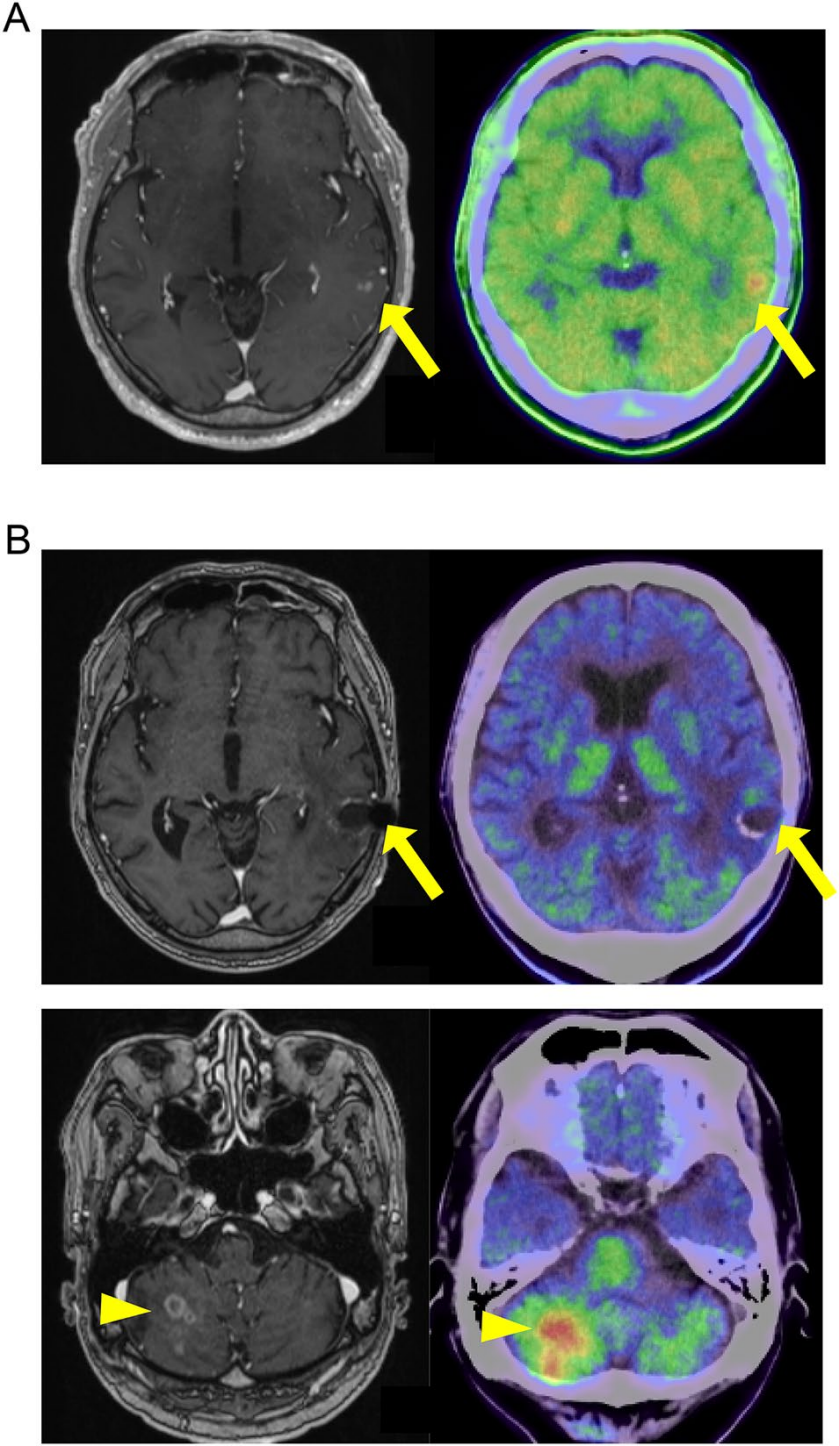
MRI and Methionine-PET images of Case 1. (A) Pre-operative MRI contrast-enhanced T1-weighted image (left) and Methionine-PET (right). Yellow arrow indicates tumor lesion. (B) MRI contrast-enhanced T1-weighted image after radiation therapy concomitant with TMZ. Yellow arrow indicates tumor lesion. (C) MRI contrast-enhanced T1-weighted image (left) and Methionine-PET (right) of the primary lesion (upper) and cerebellum (lower) on recurrence. Yellow arrows and arrow heads indicate primary lesions and recurrent lesions, respectively
